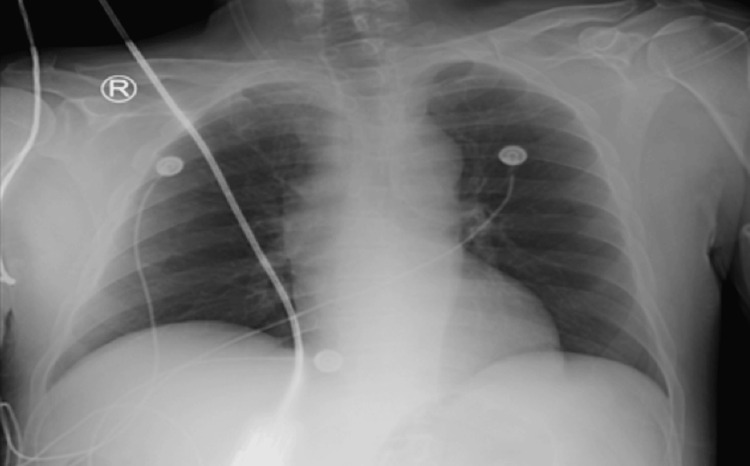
Admission chest radiograph demonstrating no abnormal findings
